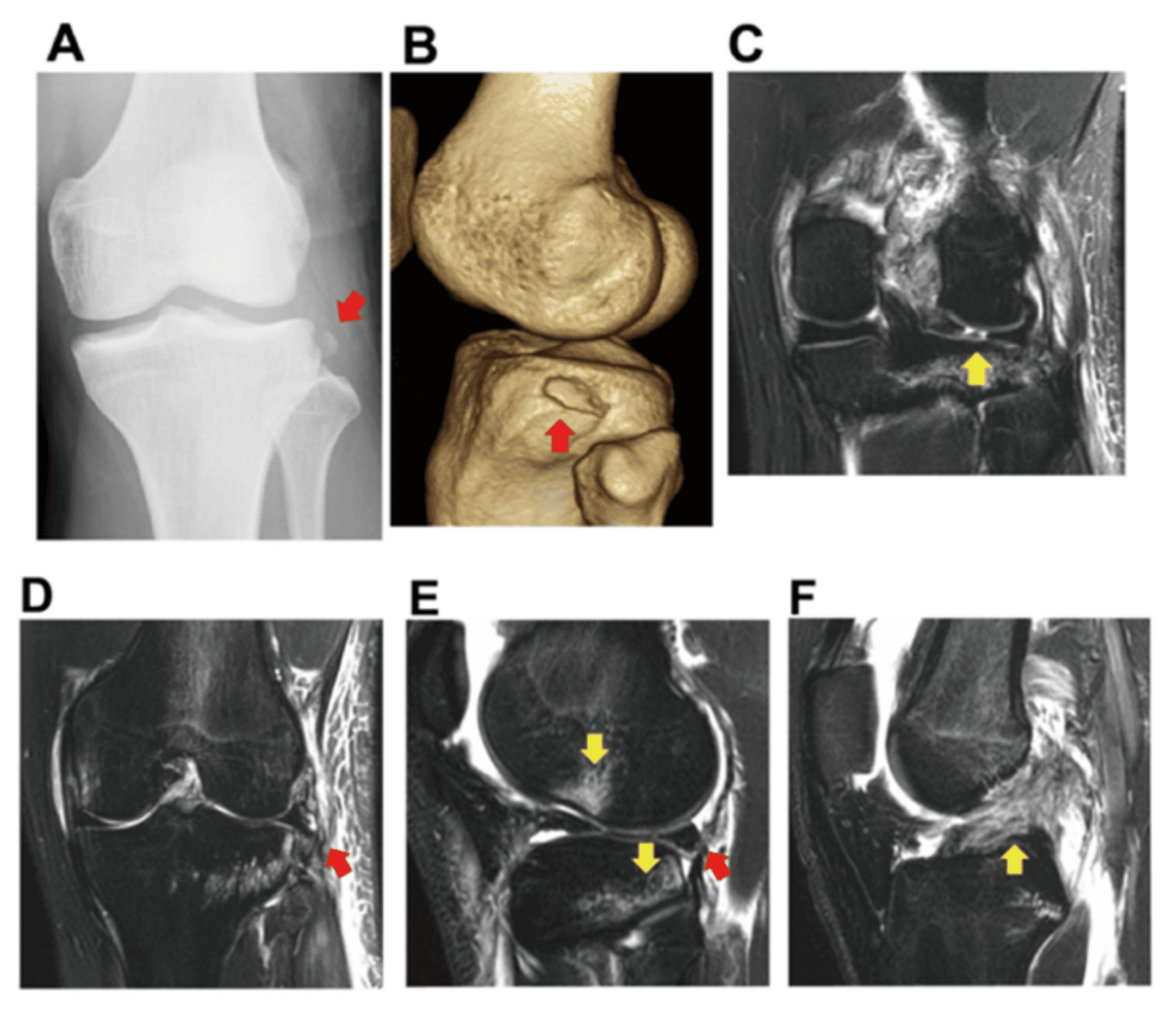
Preoperative images. (A) Anteroposterior radiograph. The red arrow indicates the Segond fracture fragment. (B) The three-dimensional CT image showing the Segond fracture fragment (red arrow). (C) The coronal view MR image showing the lateral meniscal tear in the posterior segment (yellow arrow). (D) The Segond fracture site associated with lateral extrusion of the lateral meniscus and a detachment of the joint capsule (red arrow). (E) The sagittal view MR image showing the posterior extrusion of the lateral meniscus (red arrow) and a significant bone bruise-like high-signal area on both tibial and femoral sides (yellow arrows). (F) The sagittal view MR image showing the ACL injury at the femoral attachment site (yellow arrow). CT: computed tomography; MR: magnetic resonance; ACL: anterior cruciate ligament
